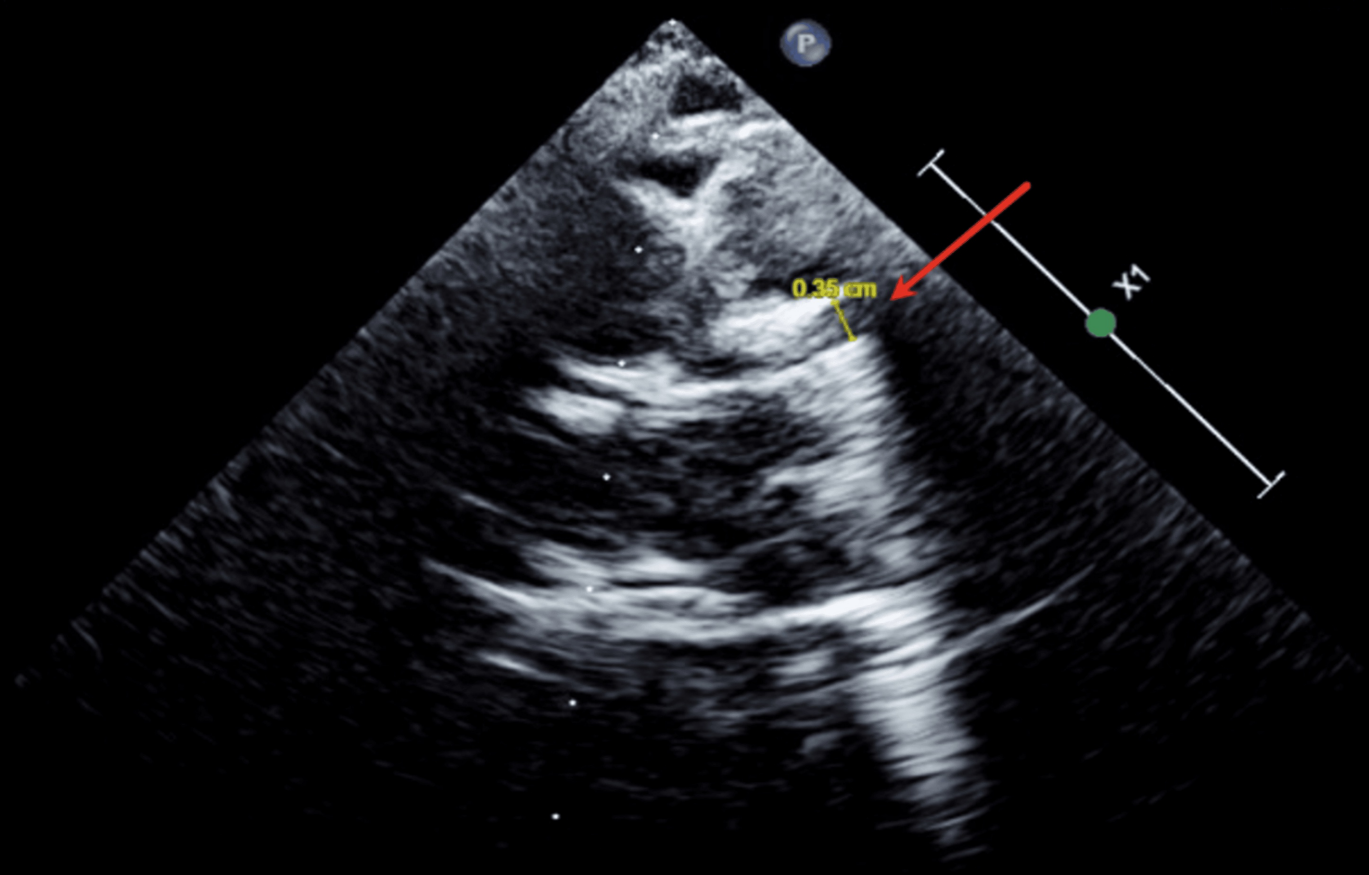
Echocardiogram from the parasternal long-axis view showing a dilated left anterior descending artery Red arrow pointing at the dilated left anterior descending artery measuring 0.35 cm
